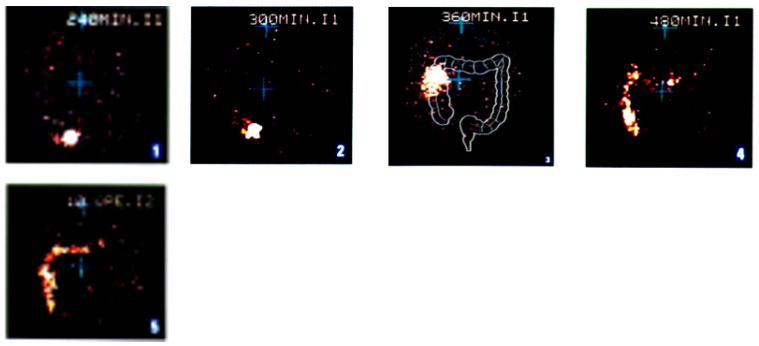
γ*-scintigraphy of double-coated *placebo* Chronotopic^®^ systems (Methocel^®^ E50 applied to 100% weight gain) relevant to subject #6.
